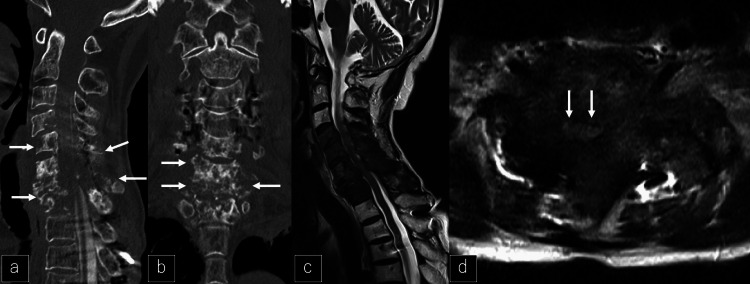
CT and MRI after discontinuation of denosumab a, b: Sagittal and coronal CT one year after discontinuation of denosumab showing bone decalcification from C4-T1 (white arrows). c, d: Sagittal and axial MRI one year after discontinuation of denosumab showing spinal cord compression (white arrows).
